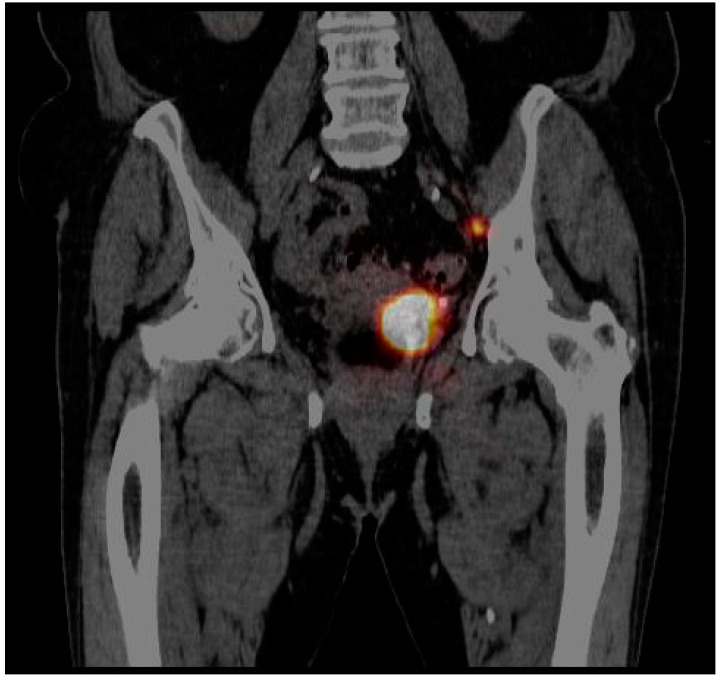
SPECT/CT example of lymphatic outflow from the left distal ureter cranially and laterally to the left outer iliac vein region.
